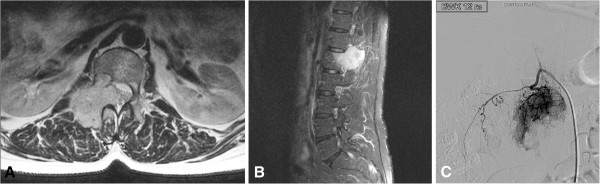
Preoperative T2-weighted axial (A) and contrast enhanced sagittal (B) MRI revealed a mass of the L1 vertebral body, originating from the right vertebral arch, extending extra- and intraspinally with a prominent mass effect to the spinal cord. Super selective catheter angiography of the bilateral spinal arteries Th11 to L2 revealed a major tumour blush from feeders originating from the right-sided TH12 spinal artery (C).
